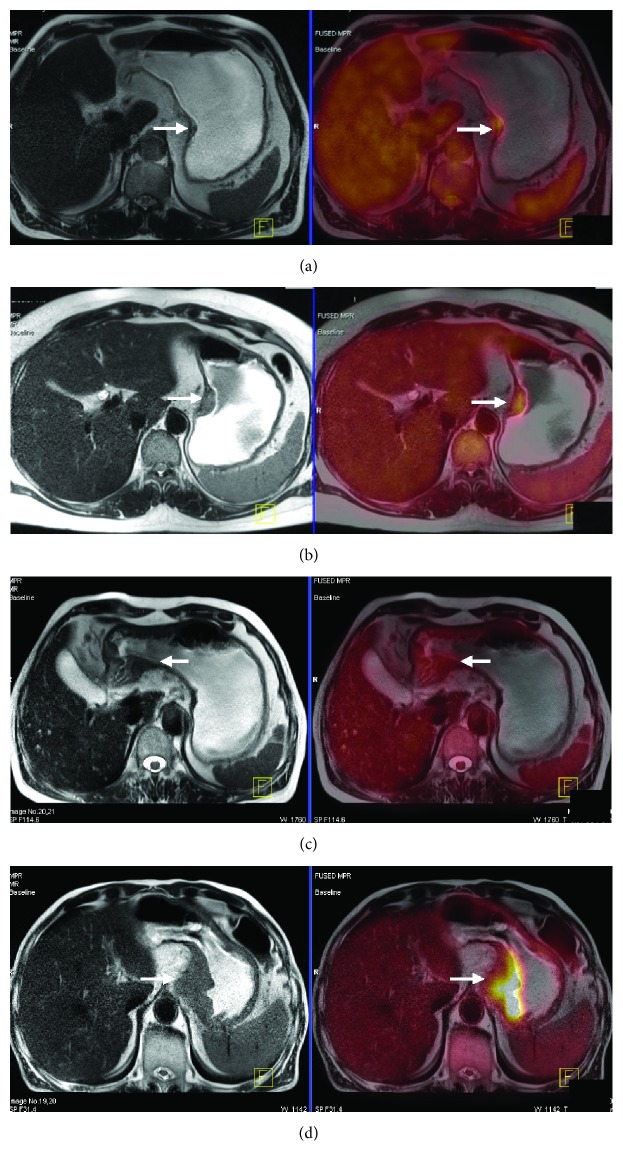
PET/MRI images of T staging. All the patients are corresponding to Figure 1. (a) A 58-year-old man with gastric carcinoma pathologically diagnosed as stage T1. Transverse T2W image shows focal thickening of the gastric wall at the lesser curvature of the stomach, the tumor of low signal intensity confined within the mucosa and submucosa, and the high signal intensity stripe of submucosa appears to be continuous (arrow). (b) A 46-year-old woman with gastric carcinoma pathologically diagnosed as stage T2. Transverse T2W image shows focal thickening of the gastric wall in the cardia with a smooth and well-defined outer border, the tumor of heterogeneous signal intensity involving the muscularis propria, and disruption of the high signal intensity stripe of submucosa (arrow). (c) A 70-year-old man with gastric carcinoma pathologically diagnosed as stage T3. Transverse T2W image shows focal thickening of the gastric wall in the antrum; the whole thickened gastric wall is infiltrated by the low signal intensity of tumor tissues with a smooth and well-defined outer border (arrow). (d) A 74-year-old man with gastric carcinoma pathologically diagnosed as stage T4. Transverse T2W image shows an irregular thickened gastric wall in the cardia and fundus; the whole thickened gastric wall with an irregular outer border is infiltrated by the low signal intensity of tumor tissues and transmural extension into perigastric fat (arrow).
